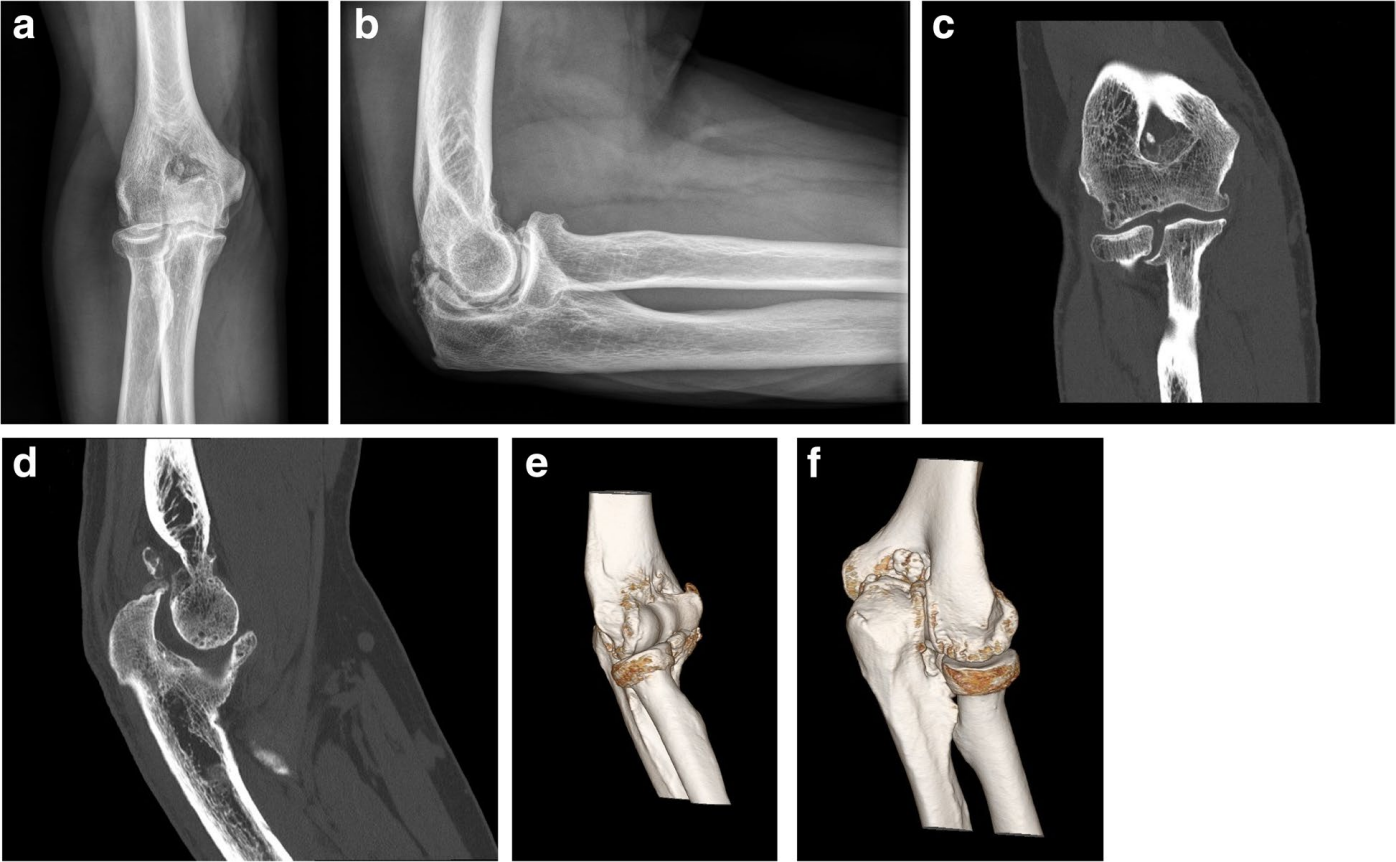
A and B Anteroposterior and lateral radiographs of an elbow with primary osteoarthritis. C – F Computed tomography with three-dimensional rendering provide is extremely useful in the evaluation of stiff elbows
